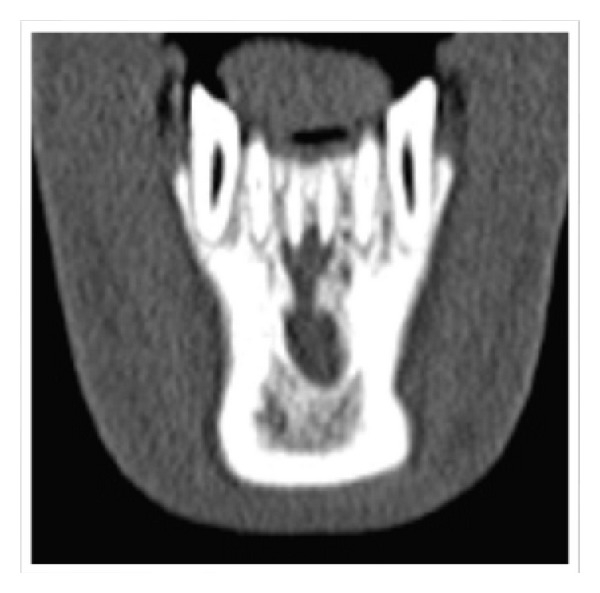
CT Image showing Dumbbell shape periapical lesion in relation to Mandibular central incisors.
